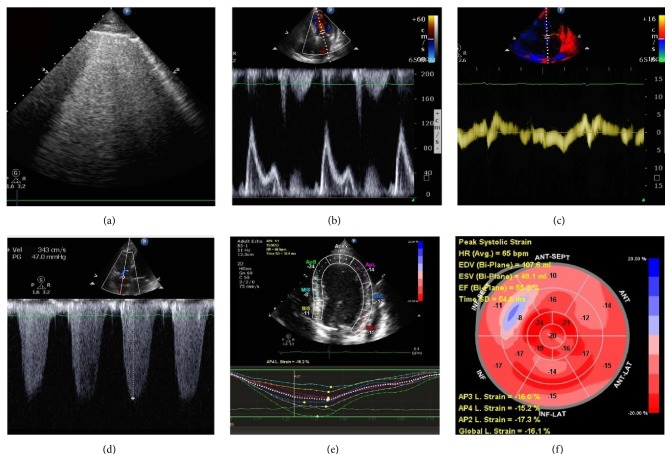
Lung ultrasound and echocardiographic parameters of a patient with congestive heart failure. (a) Interstitial-alveolar syndrome was detected by lung ultrasound. (b) Mitral inflow showed *E*/*A* > 2. (c) Tissue Doppler early (*e*′) and late (*a*′) diastolic velocities were markedly reduced. (d) Peak TR velocity by CW Doppler; peak right ventricle to right atrial systolic pressure gradient is 47 mmHg. (e, f) Global longitudinal strain analysis was −16.1%. Note that the left ventricular ejection fraction was 55.3% (f).
